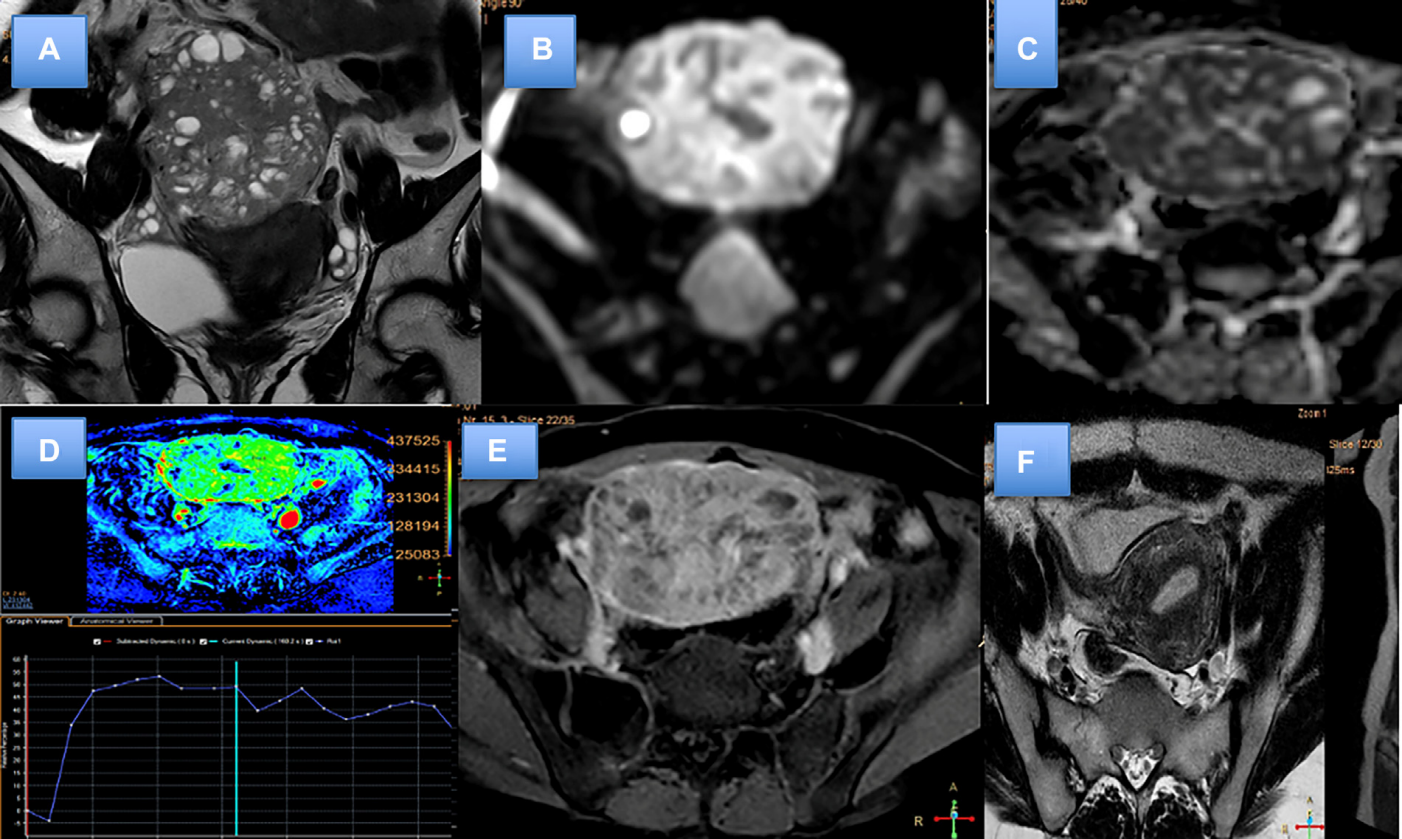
Preoperative imaging findings (A-E). MR images showed a well-defined mass in the pelvic cavity with heterogenous T2 signal intensity and interspersed cystic areas (A). diffusion restriction with high signal on DWI (B) and corresponding low signal on ADC (C). Dynamic contrast study showing type III enhancement curve (D). Heterogenous enhancement seen on post contrast study (E). Postoperative MRI (F) after 6 months showing no recurrence.
